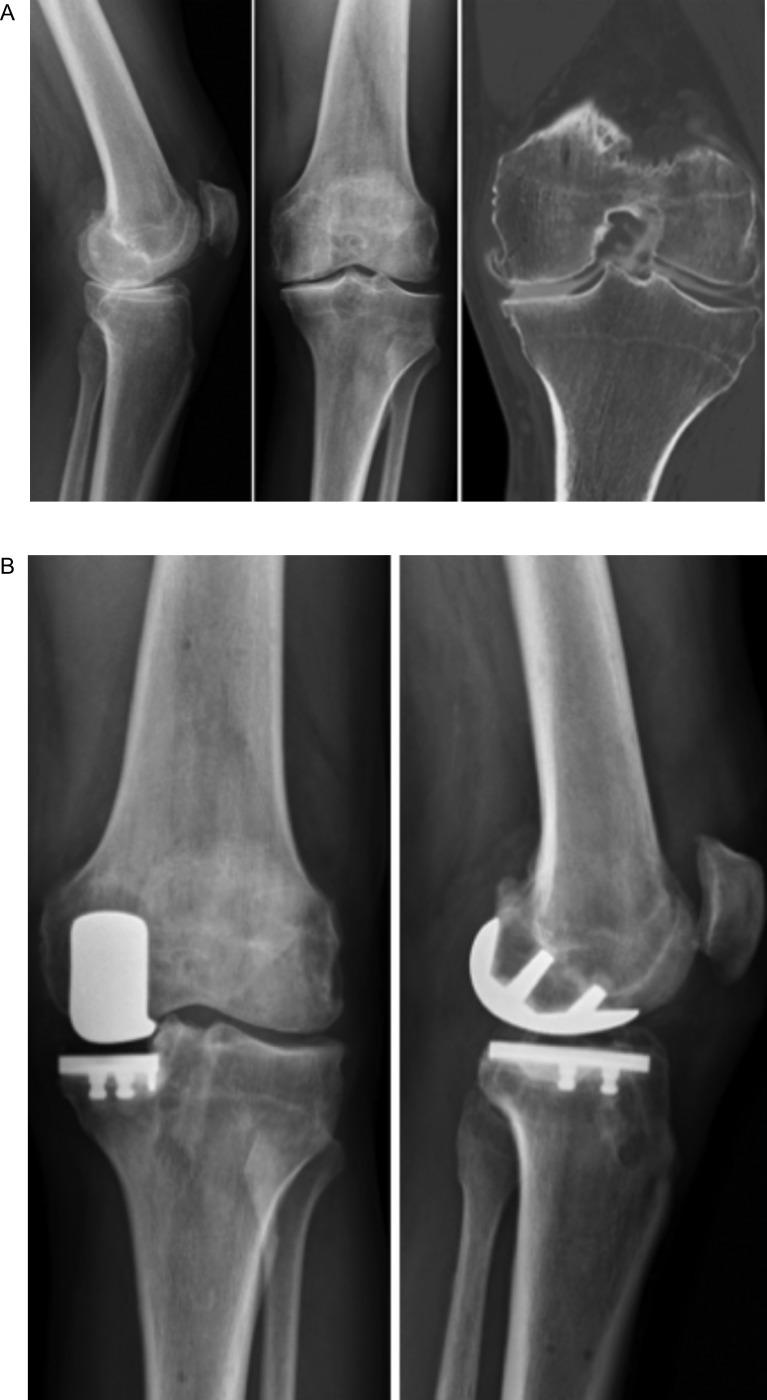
Patient of 68 years old with a prior history of medial meniscectomy, limduccited in his physical activities (cycling, highking) by medial tibiofemoral pain. The physical exam and the radiographic investigations found an anterior laxity (soft and-point to Lachman-Trillat test) and the lack of ACL with medial OA (A). An ACL reconstruction with medial UKA was performed (B). The patient was very satisfied at 18 months follow-up with no residual pain, no instability, and a complete return to sport.
